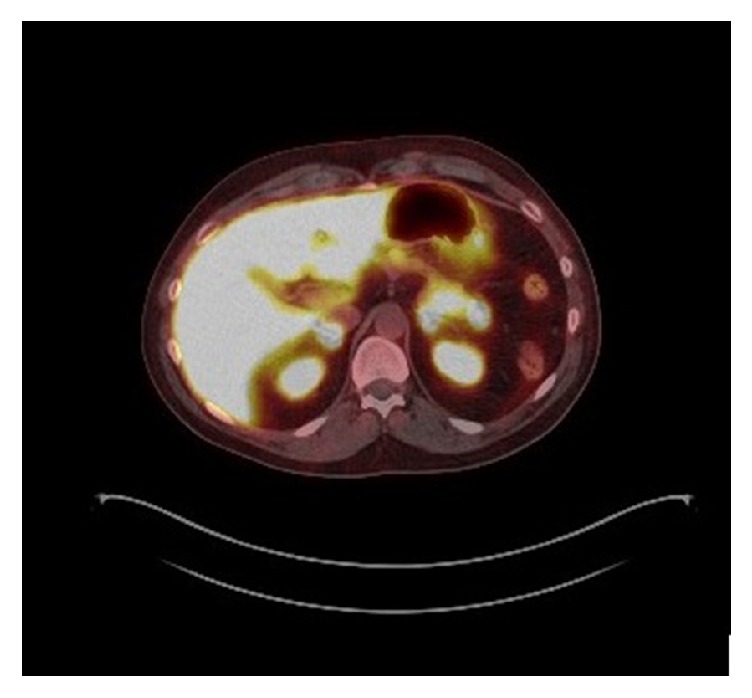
Gallium-68 DOTATATE PET/CT scan showing stability of the pancreatic tumors over time.
